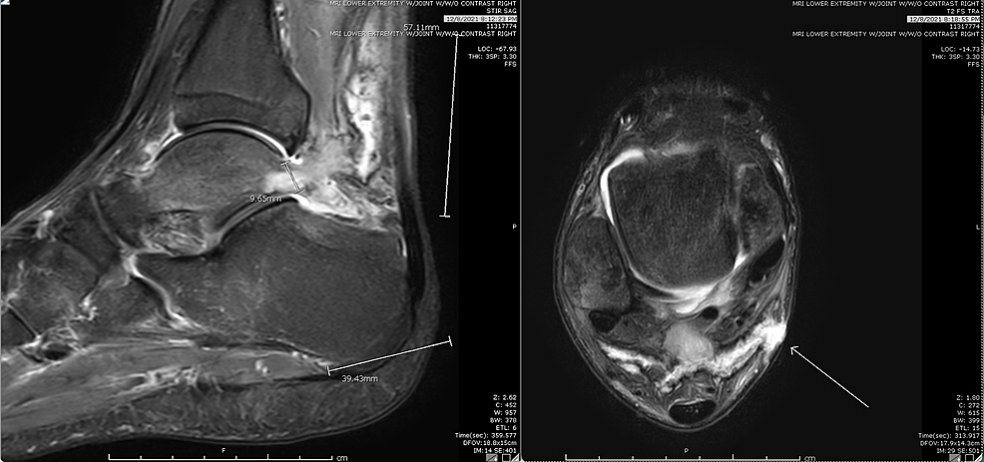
MRI of the lower extremity. A small open wound at the posteromedial aspect of the ankle with a subjacent complex rim enhancing fluid collection concerning for an abscess measuring 1.4 x 1.5 x 1.0 cm is noted. There is intraosseous penetration of this complex collection with associated erosion involving the lateral tubercle of the posterior talar process, although there is no surrounding T1 hypointense marrow replacement. There is somewhat more focally pronounced surrounding bone marrow edema-like signal noted throughout the talus as well as within the posterior subtalar facet of the calcaneus extending into the posterior superior calcaneal body, amidst a background of diffuse periarticular hyperemia/osteopenia throughout the ankle, hindfoot, and midfoot, with associated small effusions and synovitis of the ankle and posterior subtalar joints.
